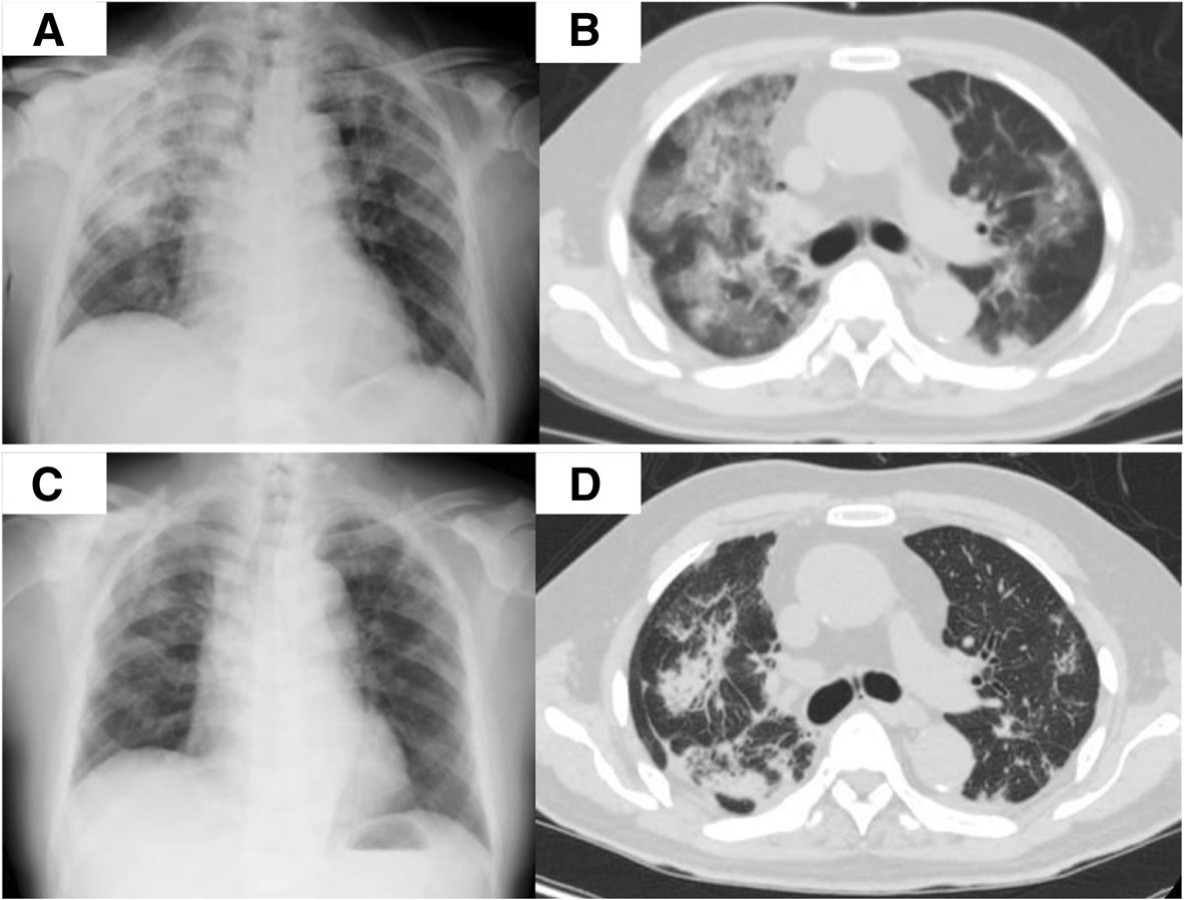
a Chest X-ray findings before the steroid pulse therapy. b Chest computed tomography findings before the steroid pulse therapy. c Chest X-ray findings after the steroid pulse therapy. d Chest computed tomography findings after the steroid pulse therapy
